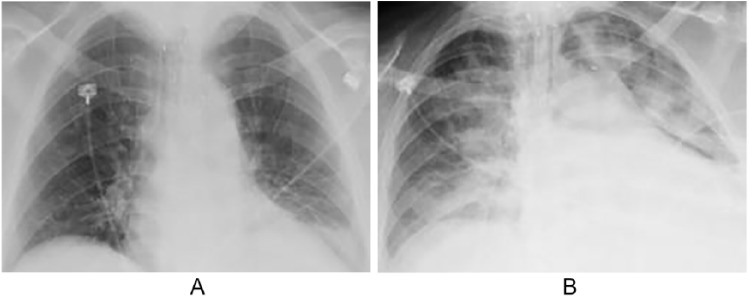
(A) Chest X-ray on admission. (B) Chest X-ray with diffuse alveolar infiltrates.
